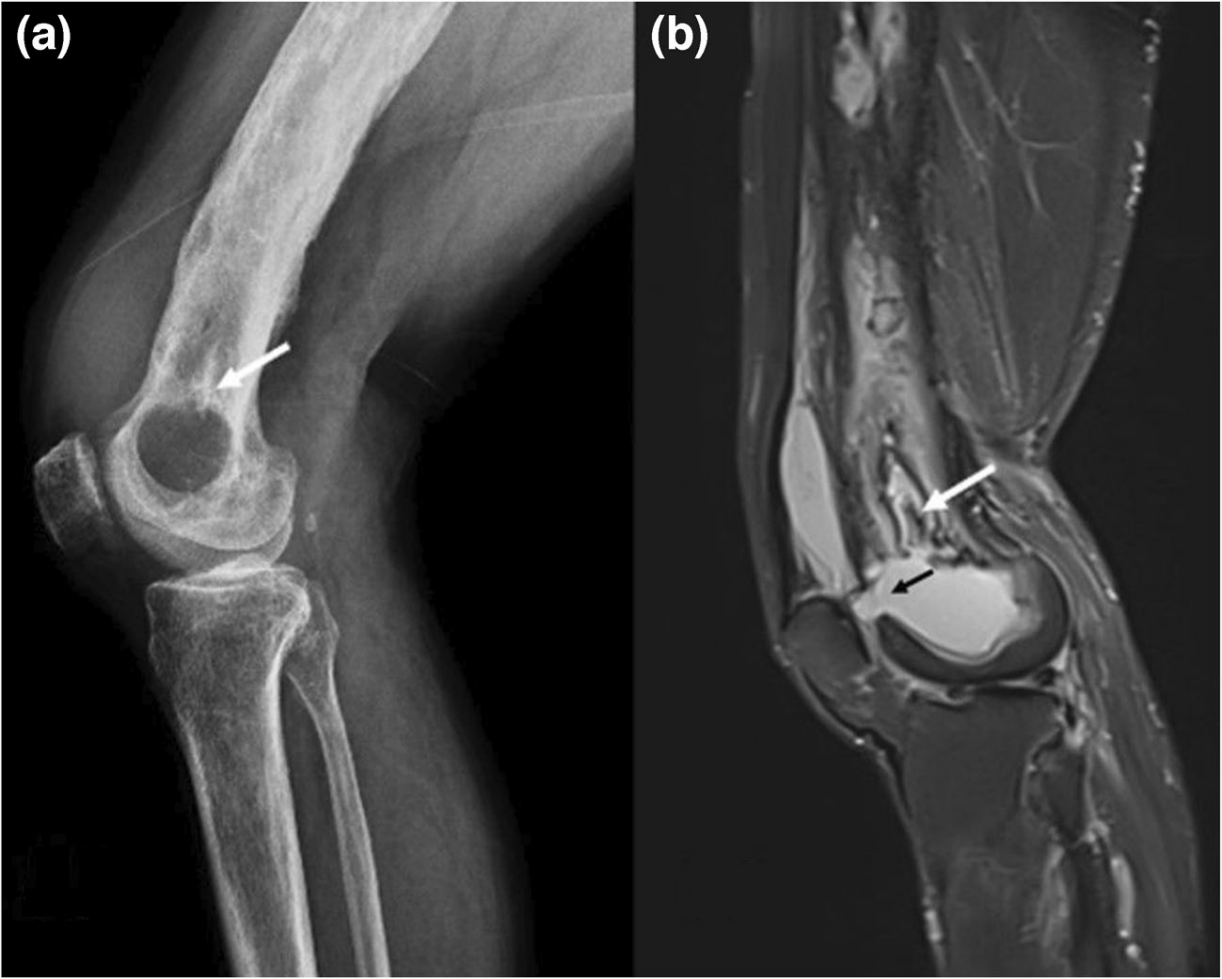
Lateral radiograph (a) and sagittal fat suppressed T2W MR image (b) of distal femur and knee joint of a 70-year-old man who suffered a trauma to the right femur 20 years ago are shown. Compatible with chronic osteomyelitis, distal femur shows sclerosis, cortical thickening and irregularity, and slight bowing deformity with a large lucent lesion in the distal metaepiphysis. The linear sclerotic lesion (white arrow) represents a sequestrum that lies immediately superior to the fluid-filled space which corresponds to the lucent lesion on the radiograph. This cystic lesion showed peripheral rim enhancement (not shown here) and represents Brodie’s abscess. Note the small defect on its anterior wall representing a cloaca (black arrow), through which the abscess cavity communicates with the suprapatellar bursa of the knee. The patient also had swelling, pain, and erythema of the knee joint compatible with septic arthritis as a complication
